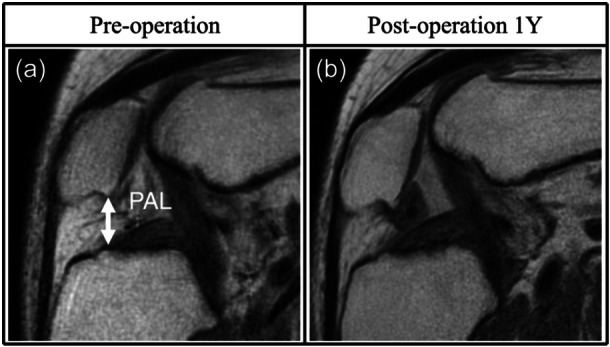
Magnetic resonance imaging findings of the postoperative reduction in patellar‐anterior cruciate ligament insertion length (PAL). (a, b) Sagittal images of the knee flexed at 90°. (a) PAL was defined as the length between the inferior edge of the patellar subchondral bone and the anterior cruciate ligament insertion, excluding osteophytes (length of the double‐headed arrow). (b) The PAL shortened postoperatively compared with that preoperatively.
